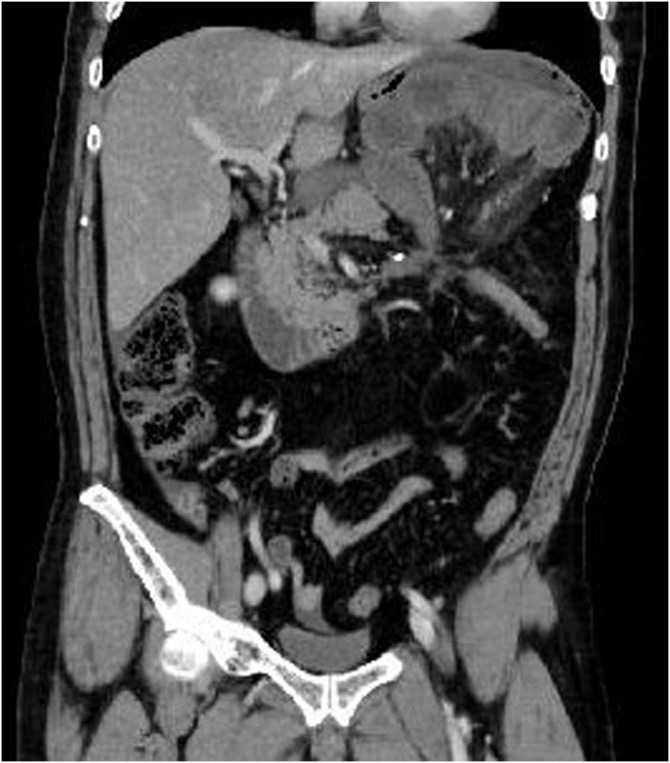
Abdominal CT scan with enhancement shows strangulated small intestine in the left upper abdomen. The strangulated intestine is superior to the transverse mesocolon.
